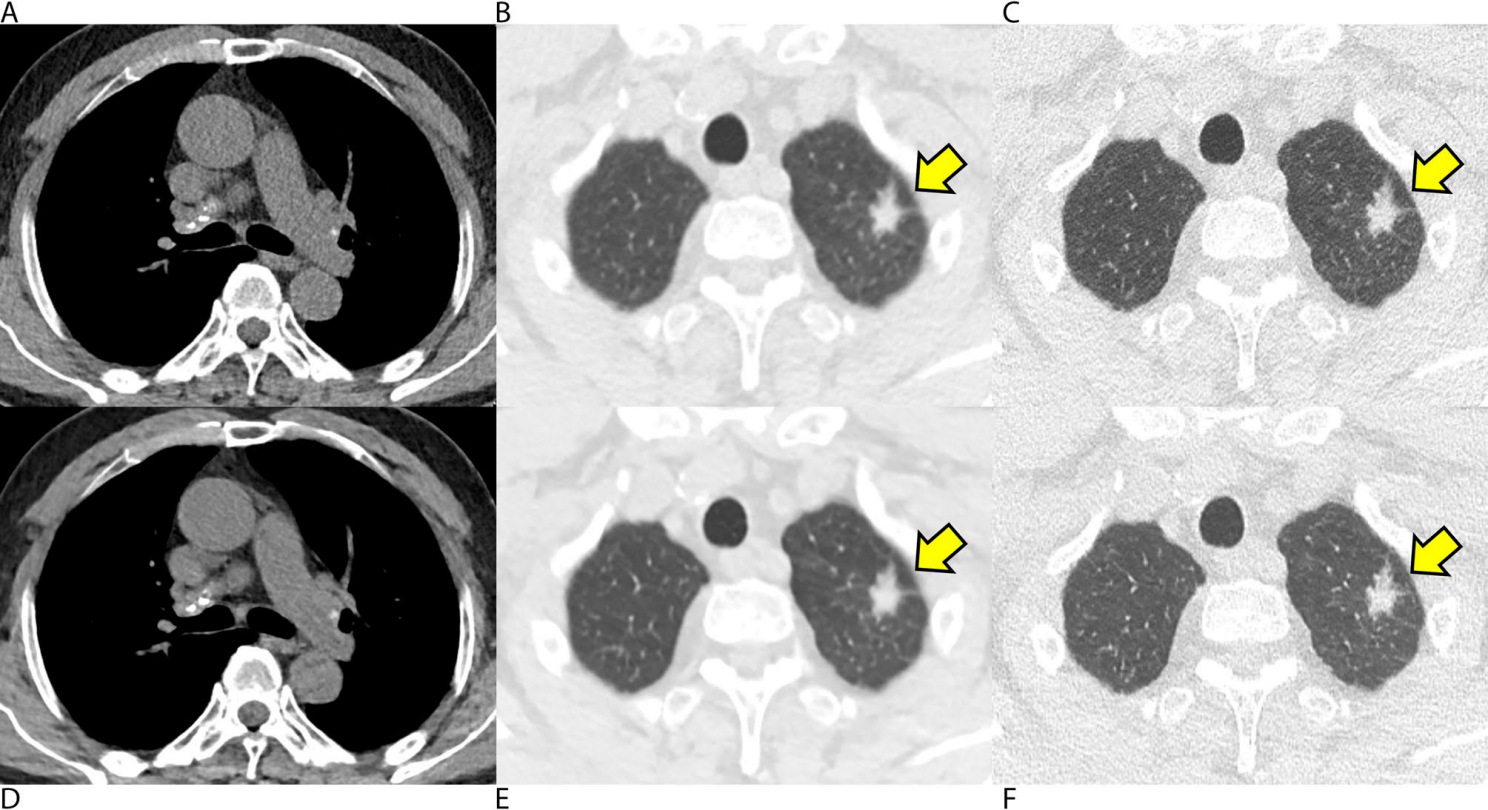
A man received low-dose chest CT for the follow-up evaluation of a spiculated lung nodule. (A-C) Conventional low-dose chest CT images were reconstructed with iterative reconstruction, and (D-F) the images generated using a quarter dose of radiation were reconstructed with commercial deep-learning software. (A, D) Soft-tissue structures including calcified mediastinal lymph nodes were visualized with a lower noise level in the mediastinal-window setting on both images. (C, F) A spiculated nodule in the left upper lobe was well visualized with a sharp margin on both images (arrow)
